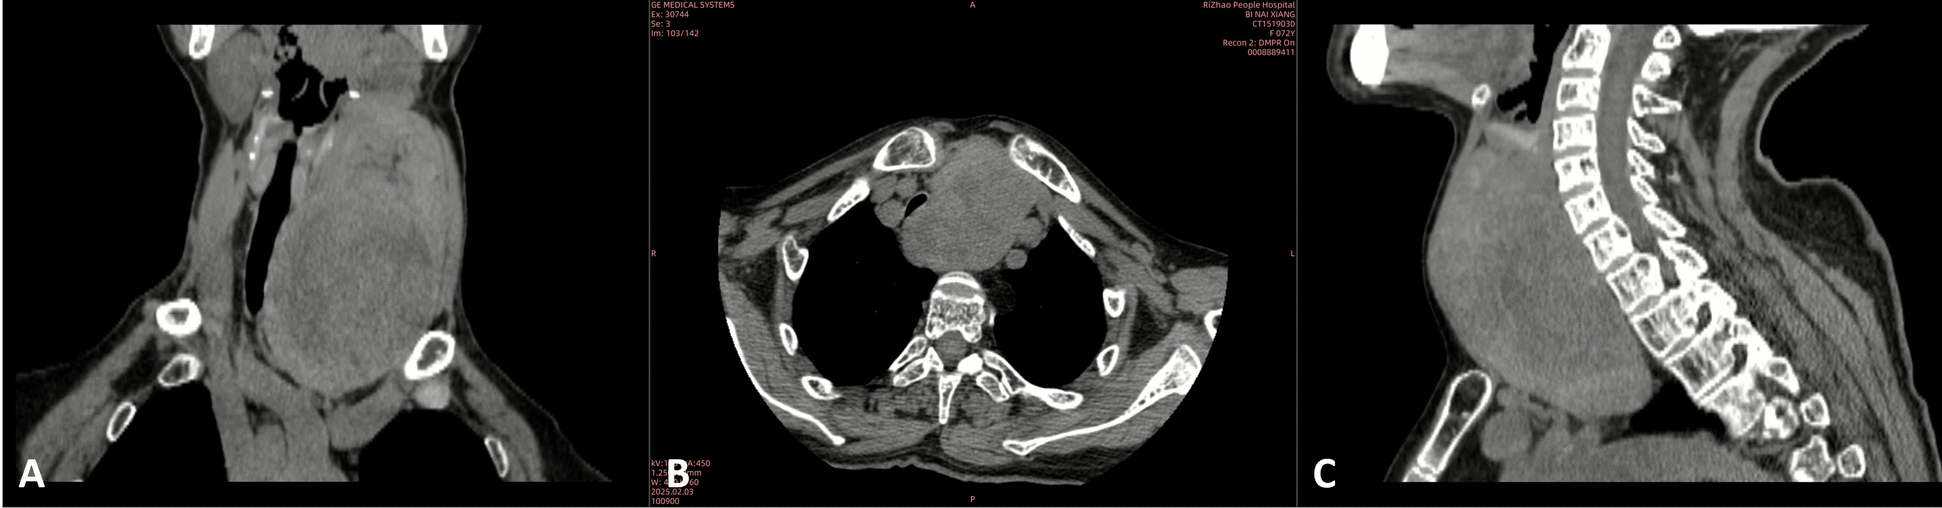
Contrast-enhanced CT images of the neck demonstrating a large mass causing compression of the surrounding structures in different planes, including coronal (A), axial (B), and sagittal (C) views
